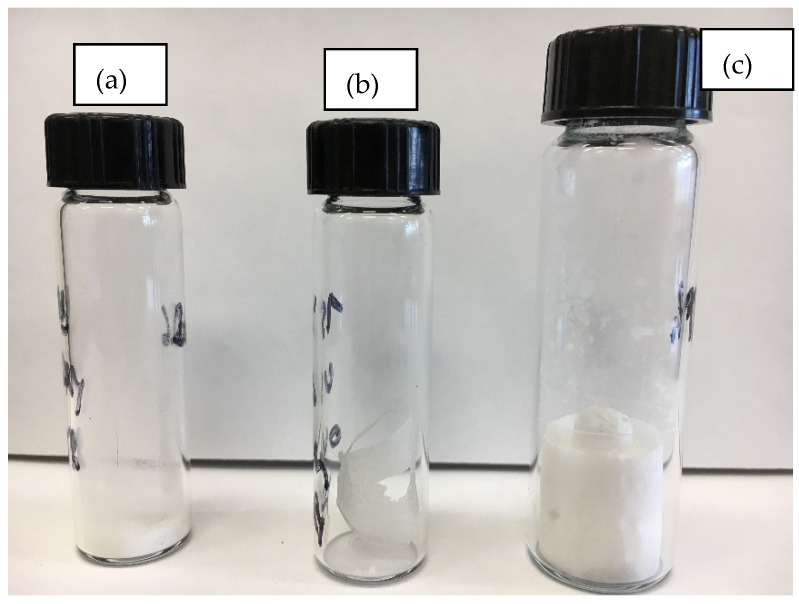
Different macro-morphologies of the potato starch from “Gabrielle” cultivar after treatment using ultrasonication under dry conditions (a); ultrasonication under wet conditions followed by drying in a vacuum oven at 40 °C (b); ultrasonication under wet conditions followed by drying by lyophilization (c).
